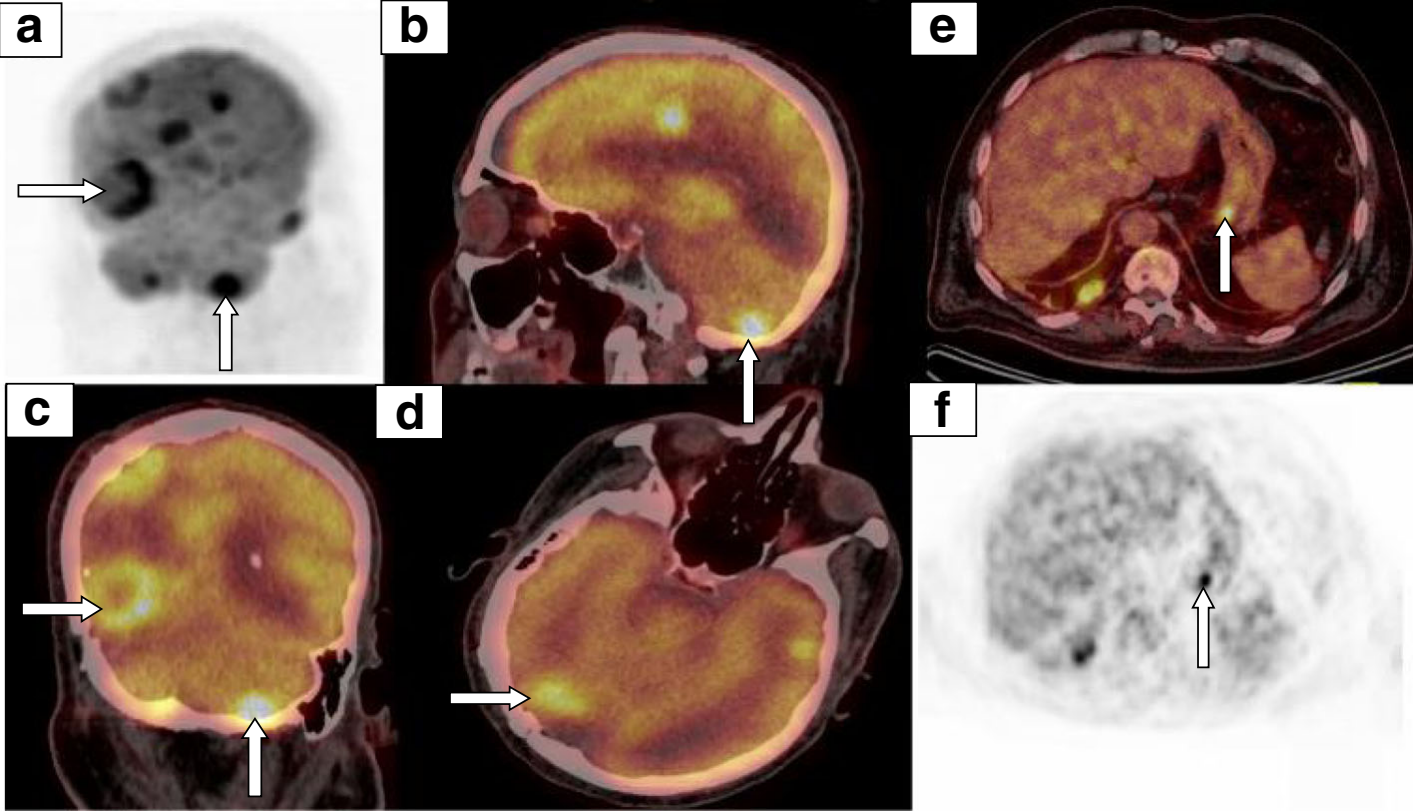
^18^FDG TEP TDM. a, b, c, d Hypermetabolic cerebral and cerebellar lesions related to secondary lesion of malignant meningioma. e, f Hypermetabolic lesion at the fundus
